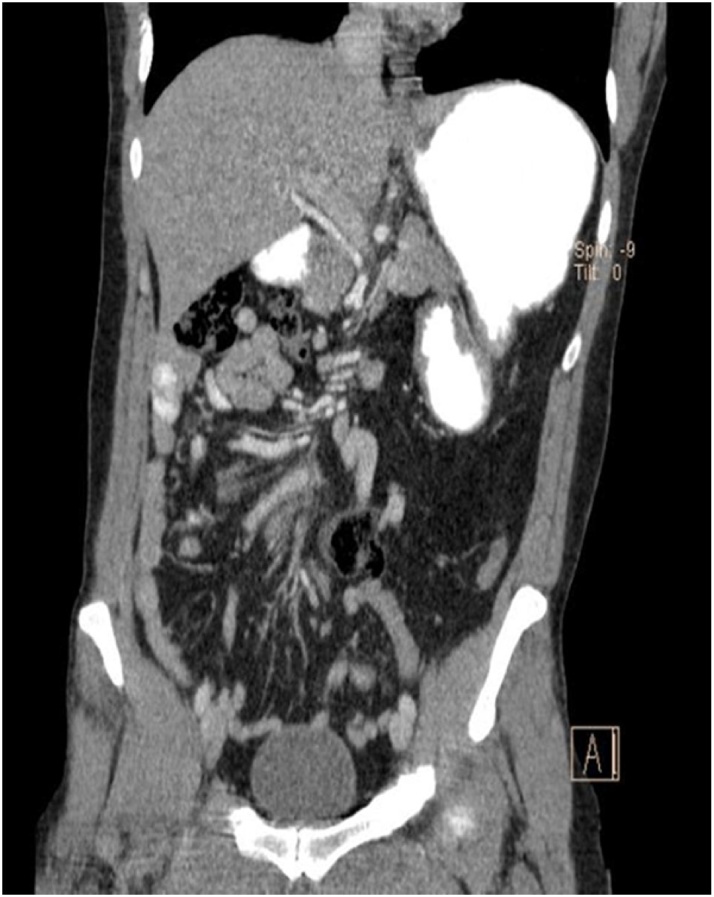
Spiral CT Scan demonstrates midgut malrotation with Volvulus in coronal view.
